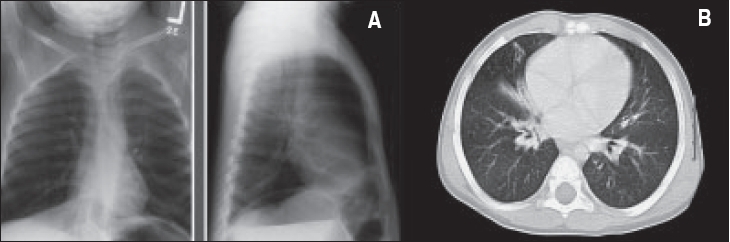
A patient with RML syndrome. A. Chest X-ray: Shows generalized
